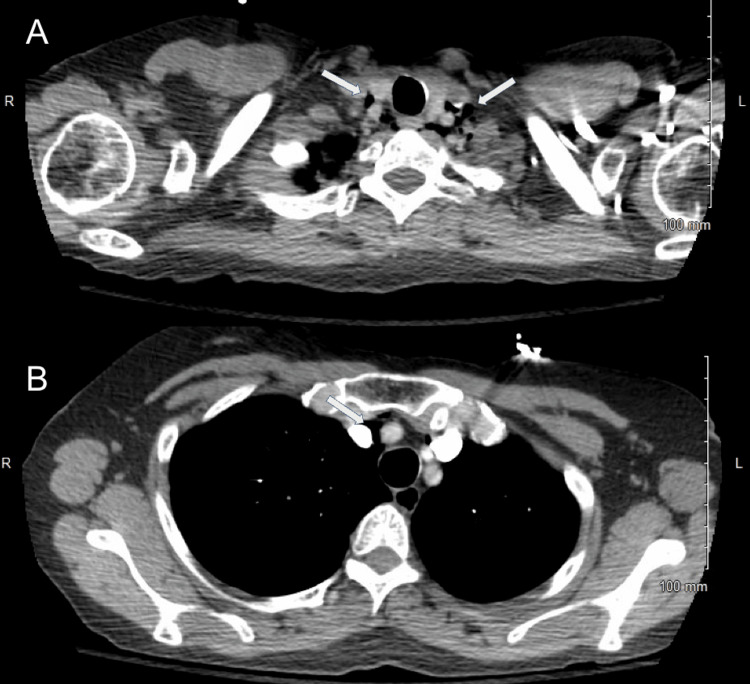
CT of the chest demonstrating PM (A) Superior axial slice showing air in the anterior mediastinum. (B) Inferior axial slice also demonstrating anterior mediastinal air without pneumothorax. PM, pneumomediastinum
